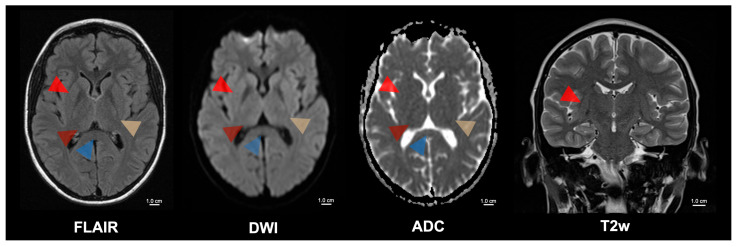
Five-month follow-up of the patient with cerebral malaria. Cerebral malaria is associated with a high mortality rate. However, those patients who survive often have a complete recovery with minimal or no long-term sequelae. Despite the formerly extensive MRI changes, the patient had a complete motor and cognitive recovery after treatment with artesunate and artemether/lumefantrine. The follow-up MRI of the presented patient showed a complete regression of the previously observed pathological signal changes in the brain parenchyma. These signal changes were formerly present in the internal capsule (yellow arrowhead), the basal ganglia (bright red arrowhead), the thalamus (dark red arrowhead), the mesencephalon, the subthalamic nuclei, and the splenium corporis callosi (blue arrowhead). Supplementary Table S1 contains an overview of the patient’s progress over time, including the date of infection, the start of treatment, the antimalarial drugs used (artesunate and artemether/lumefantrine), the dosages and the date of cure. Rasalkar et al. detailed four cases in which MRI consistently revealed bilateral thalamic infarctions, with or without hemorrhage; one patient additionally had acute hemorrhagic infarctions in the brainstem and cerebellum and subsequently died, whereas the other three survived with antimalarial therapy, and follow-up scans showed resolution of the thalamic lesions [8]. In patients returning from malaria-endemic regions, any abrupt neurological deterioration must prompt an immediate malaria diagnosis, as malaria treatment has been shown to reverse changes in motor and cognitive function, and reverse striking MRI abnormalities, thereby allowing full neurological recovery in some cases. FLAIR = Fluid-attenuated inversion recovery; DWI = Diffusion-weighted magnetic resonance imaging; ADC = Apparent diffusion coefficient; T2w = T2-weighted; cm = centimeter.
